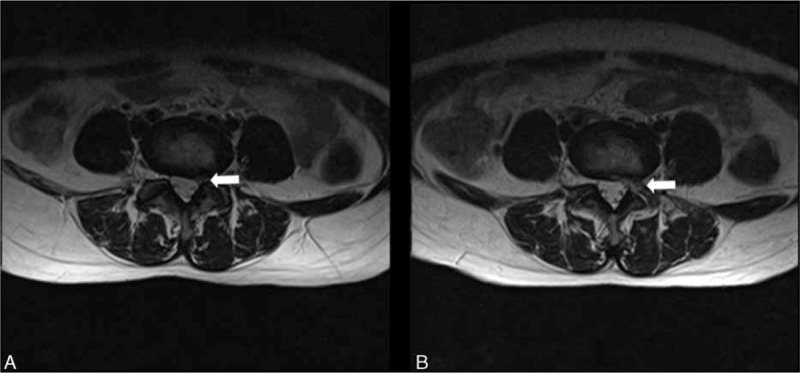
A representative patient with L4/5 left disc herniation who underwent PELD by the conventional foraminoplasty. In picture A, it demonstrates that the extruded nucleus pulposus defects the left L5 nerve root in the epidural space before surgery (white arrow). The postoperative axial MRI findings enable to detect not only the complete removal of disc fragments, but also the damage of articular facet in picture B (white arrow). MRI = magnetic resonance imaging, PELD = percutaneous endoscopic lumbar discectomy.
